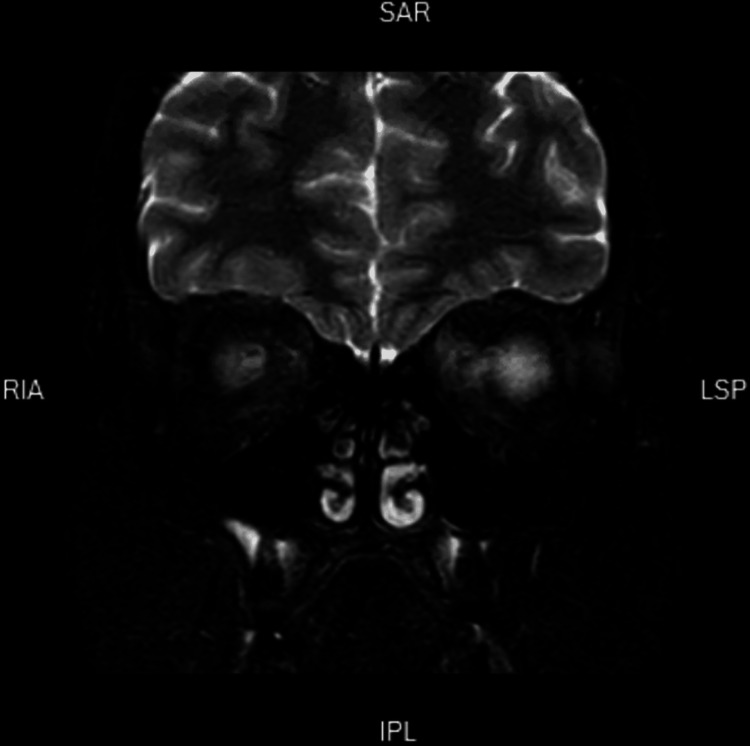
T2 brain MRI (coronal section) demonstrates enhancement of the pre-chiasmatic segment of the left optic nerve.
